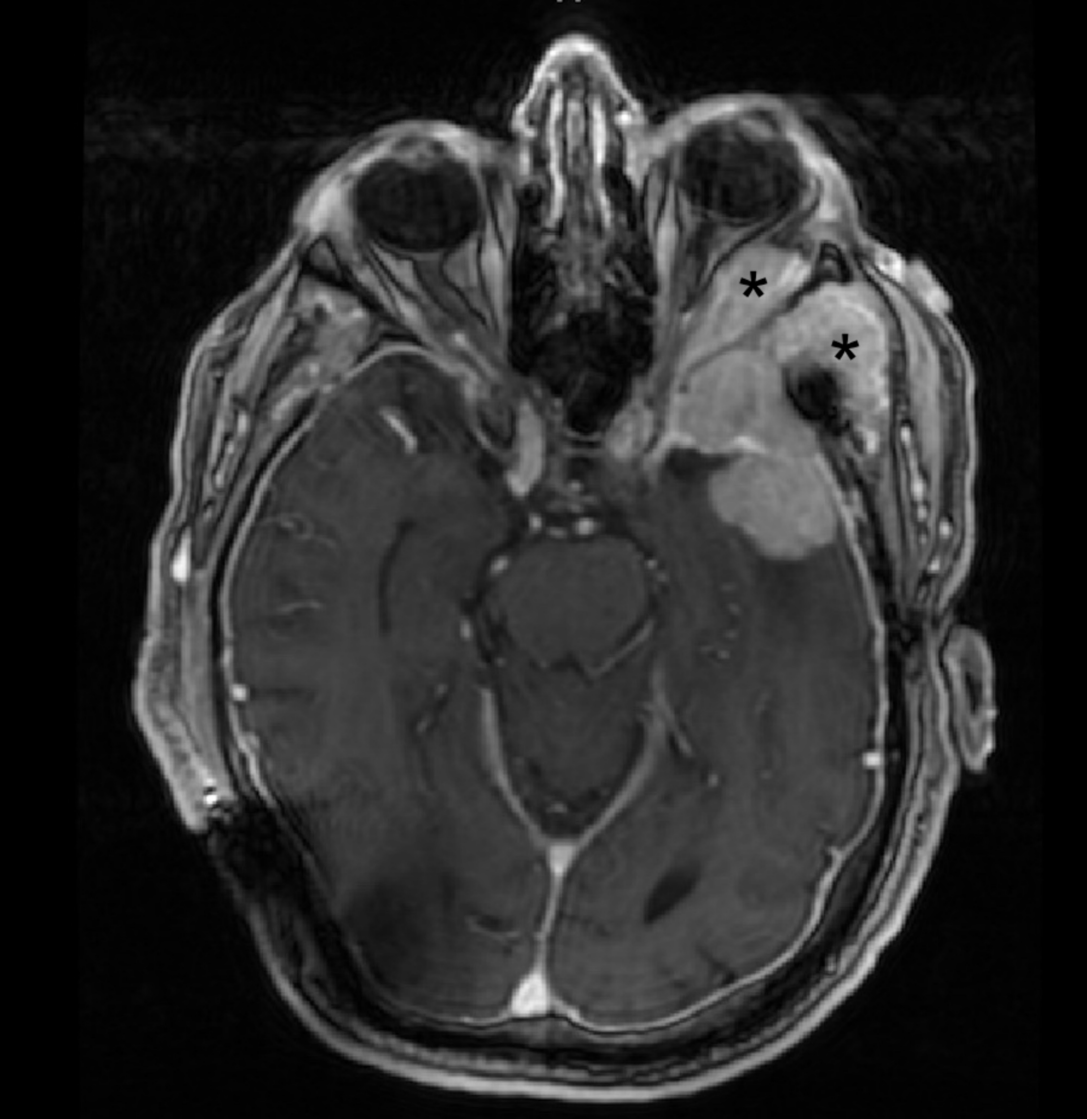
Preoperative axial MRI demonstrating tumor involvement of the lateral orbit and temporalis muscle * = Tumor invasion of the lateral orbit and temporalis muscle MRI: magnetic resonance imaging
